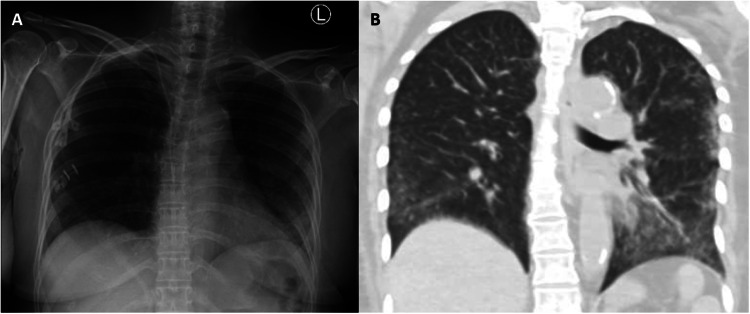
Chest radiology of a patient with disseminated toxoplasmosis. (A) Chest radiograph with diffuse bilateral peripheral infiltrates and (B) Computed tomography of the chest showing diffuse bilateral ground glass opacities consistent with bilateral pneumonitis.
